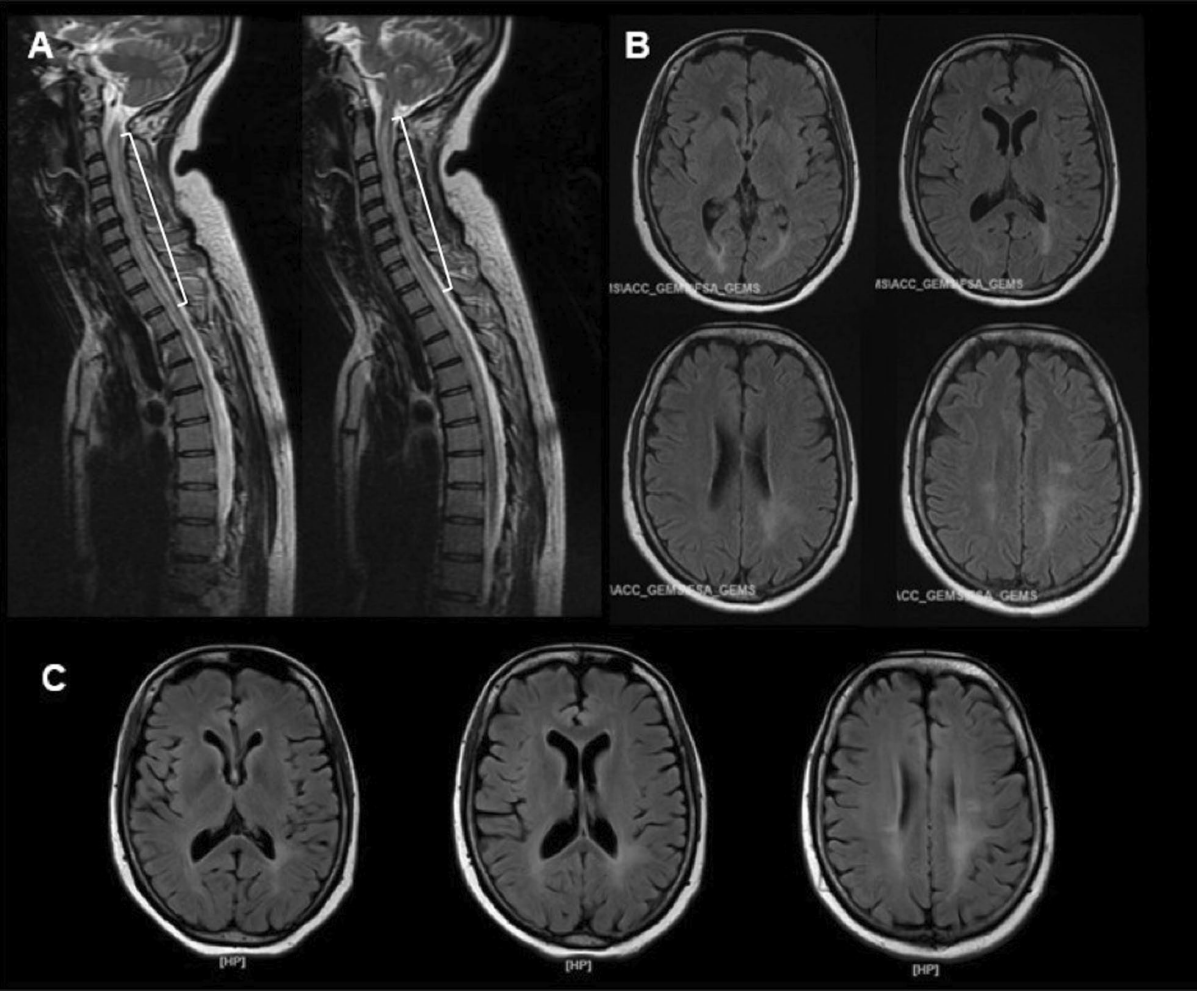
A Spine MRI scan before the first admission, showing T2 hyperintensity along the cervicothoracic region, corresponding with LETM; B brain MRI result during the first admission, revealing hyperintensity in T2 and fluid-attenuated inversion recovery (FLAIR) in the posterior horn of the lateral ventricles; C repeat brain MRI scan obtained during the second admission, exhibiting no new lesion
